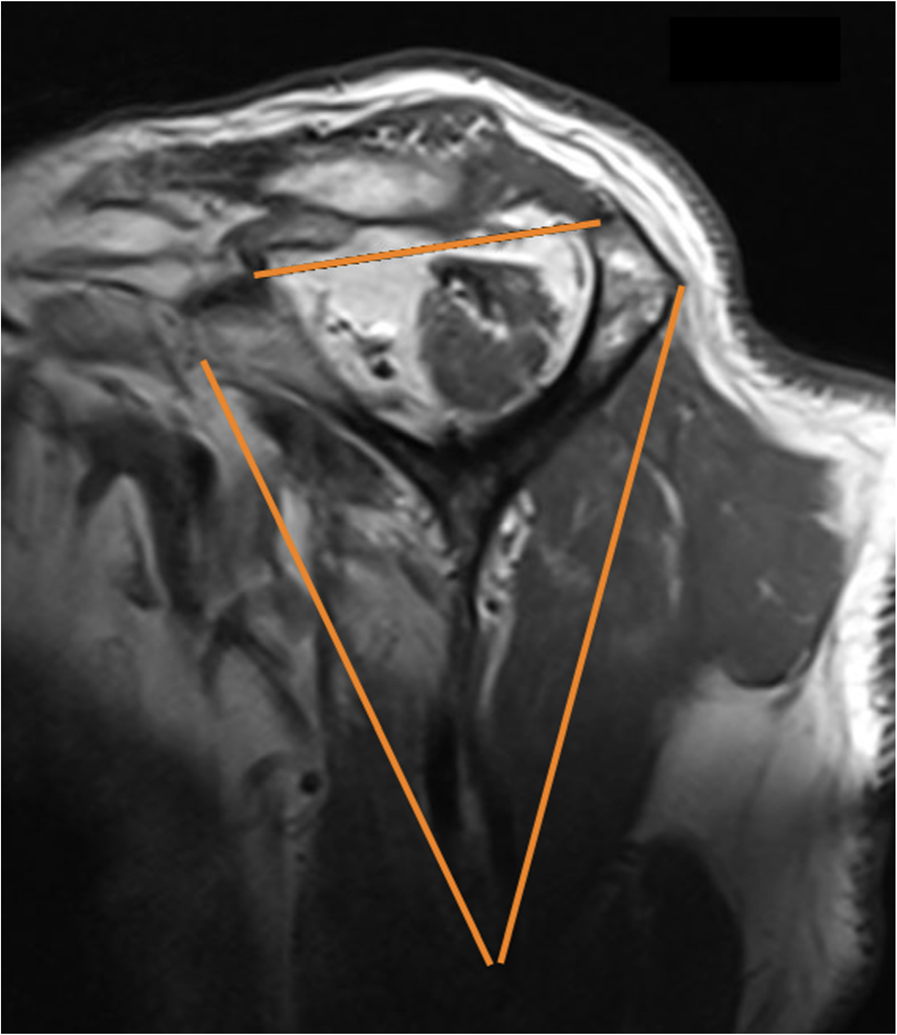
From the sagittal oblique view of T1-weighted magnetic resonance imaging, the muscle atrophy were evaluated in the most lateral image on which the scapular spine was in contact with scapular body (Y - shaped view). The Zanetti’s tangent line extended from superior aspect of the coracoid to the superior border of the scapular spine, then the other two lines were extended from each scapular process to tip of scapular body
